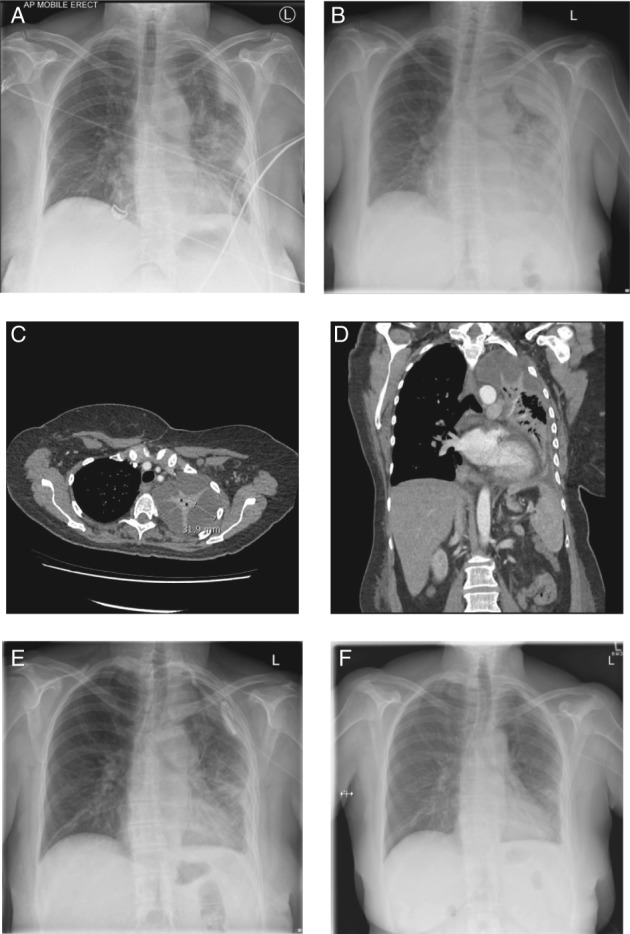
Chest X‐ray and computed tomography (CT) images during treatment. (A) Day 1 – left lower zone consolidation and left upper zone loculated pleural effusion; (B) day 9 – increase in size of left loculated pleural effusion; (C) and (D) day 7 CT – left apical loculated fluid collection; (E) day 12 – persistent loculated pleural effusion with intercostal catheter in situ prior to tissue plasminogen activator (tPA)/deoxyribonuclease (DNase) treatment. (F) Five days after completion of tPA/DNAse treatment.
